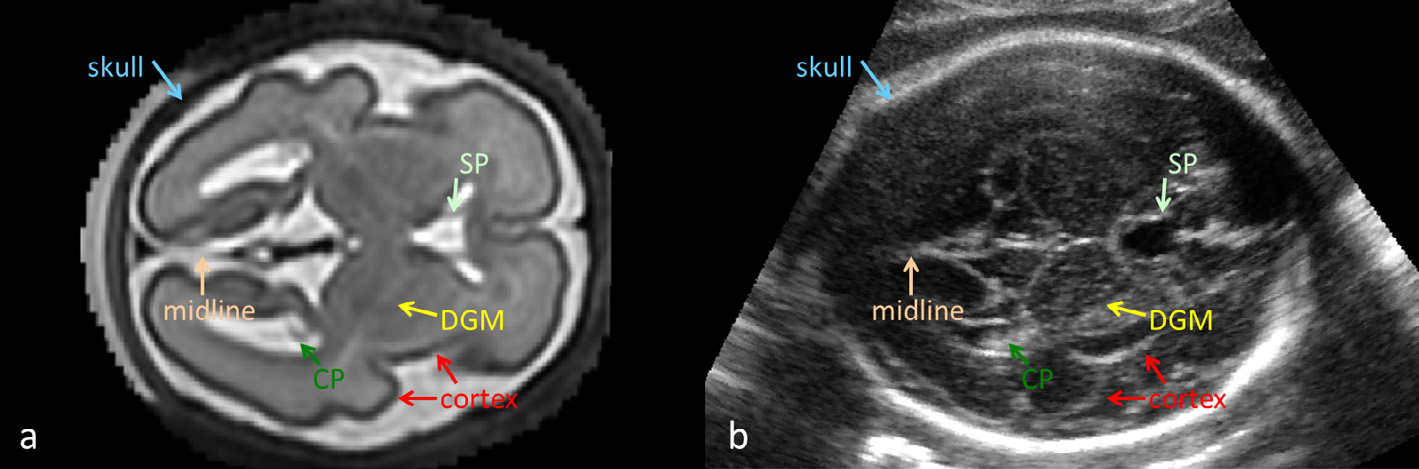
Features visible in fetal brain US in axial view: skull, cortical surface, midline (falx), DGM, choroid plexus (CP) and septum pellucidum (SP). Positions of these features in MRI (a) and in US (b). The MR and US images presented here are scans of different subjects with similar GA.
